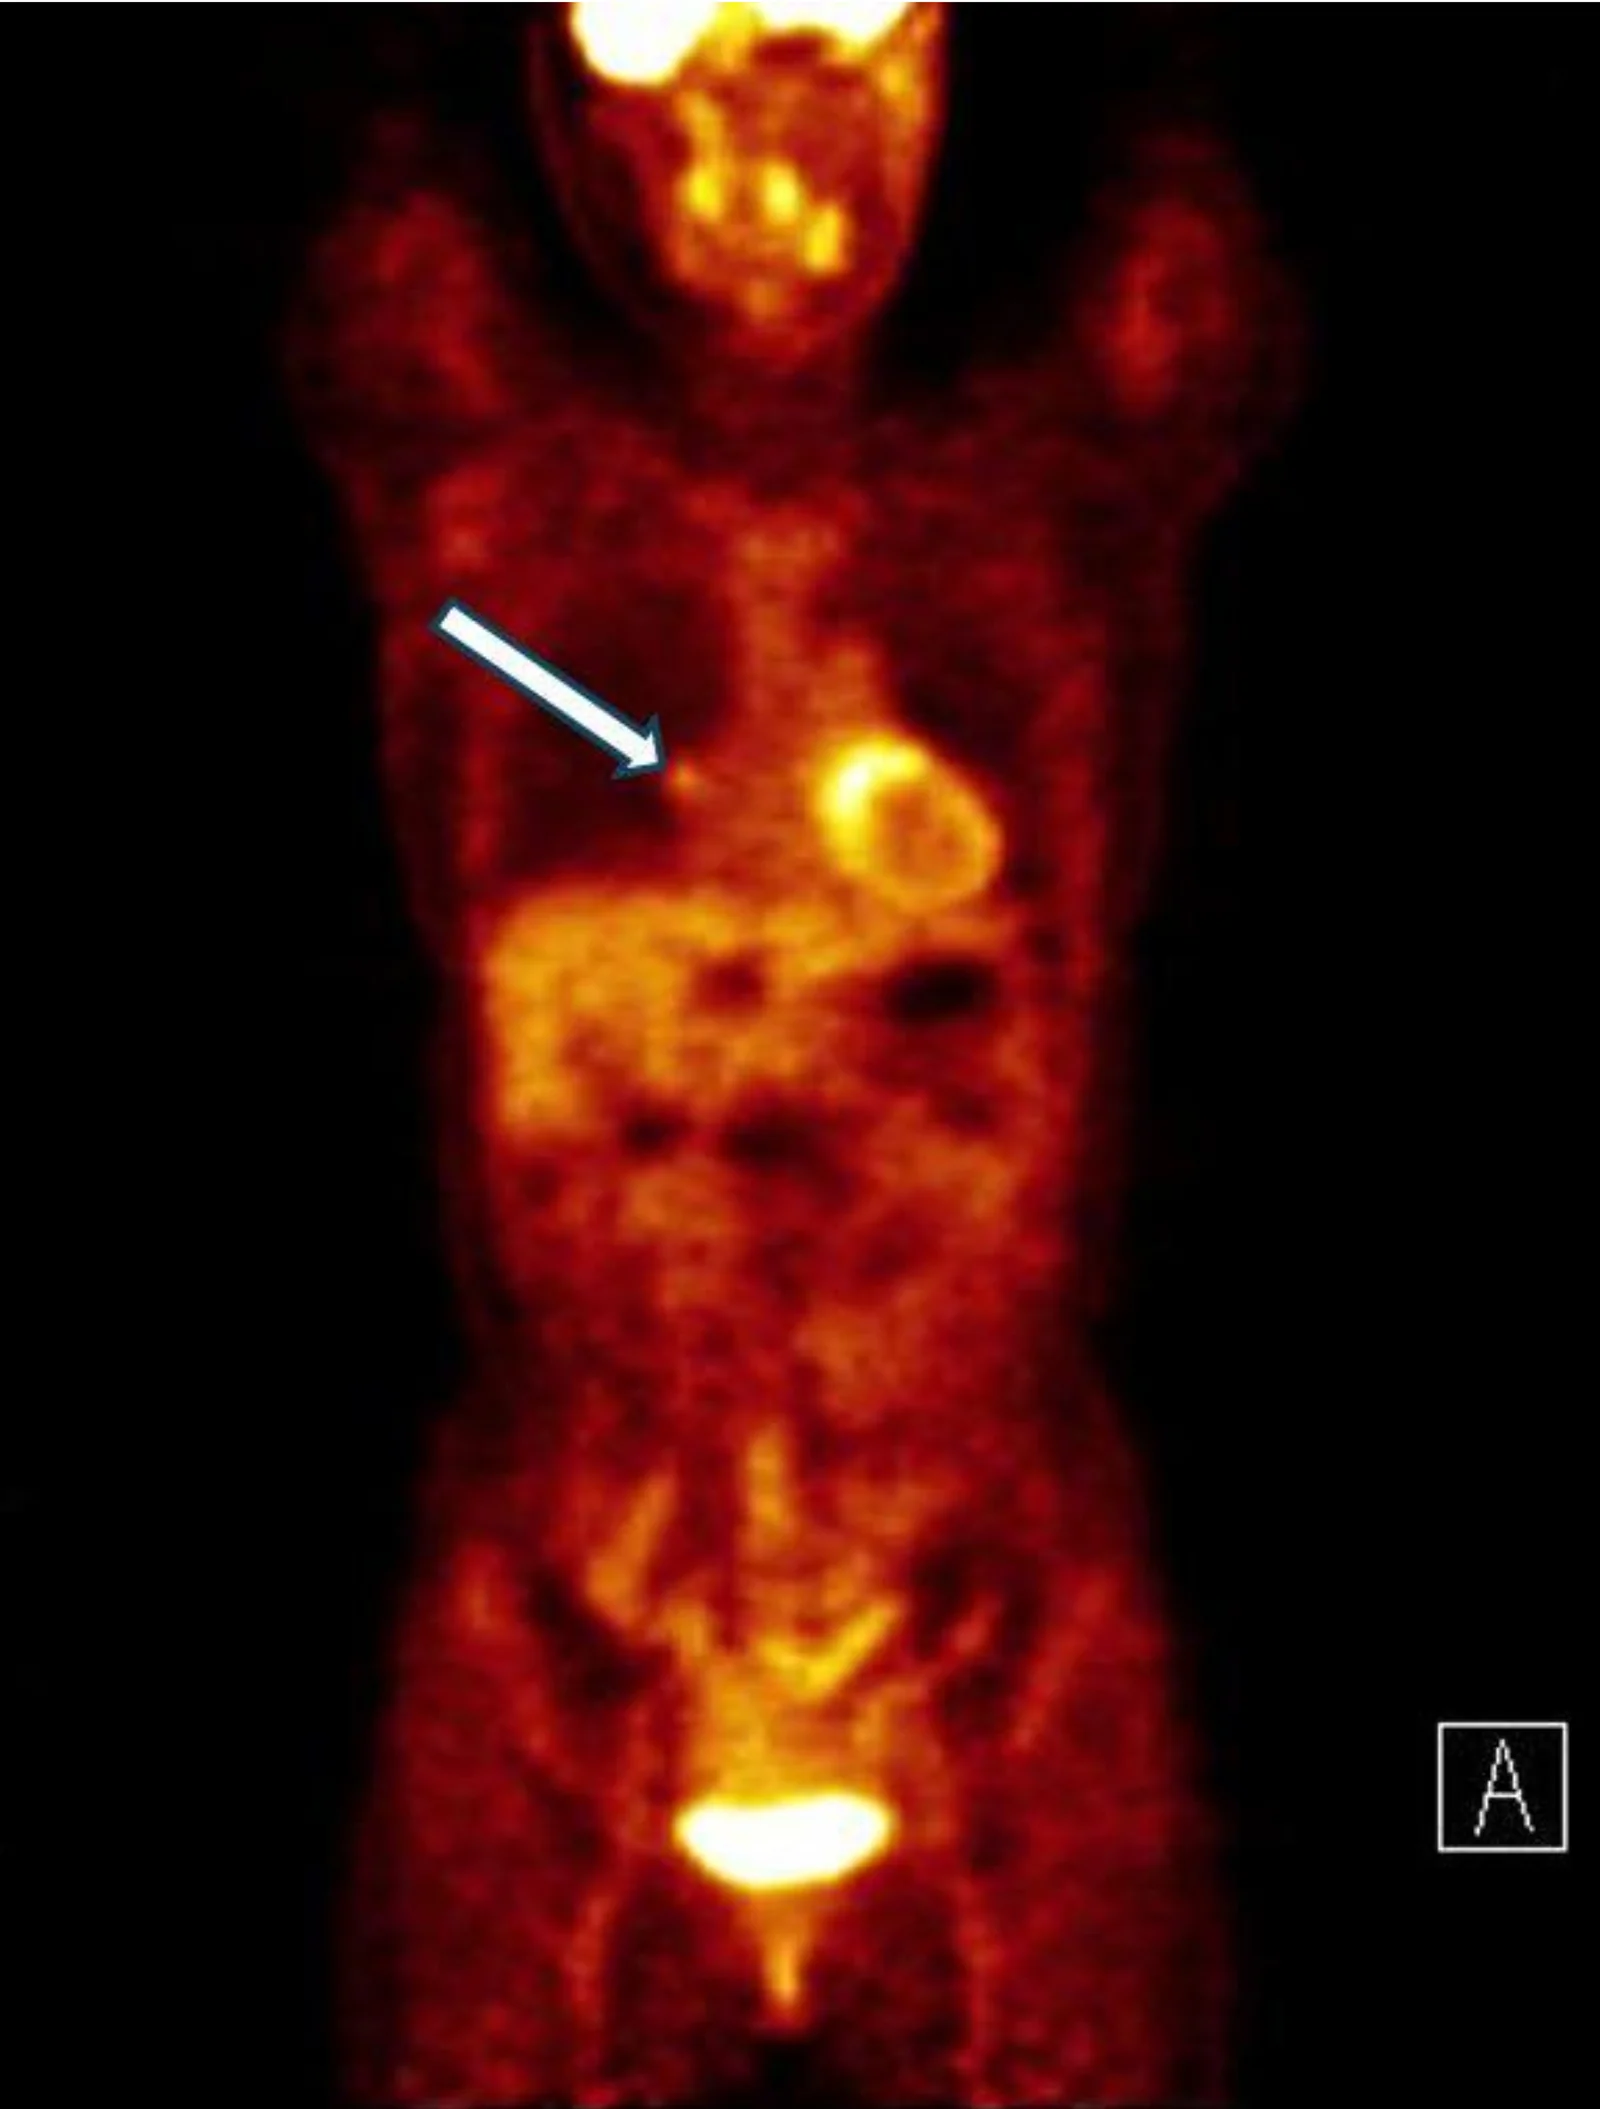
PET/CT showed a nodule (arrow) with mild FDG uptake with a maximum standardized uptake value (SUVmax) of 1.6 in the right middle lobe. CT = computed tomography, FDG = F-fluorodeoxyglucose, PET = positron emission tomography.
